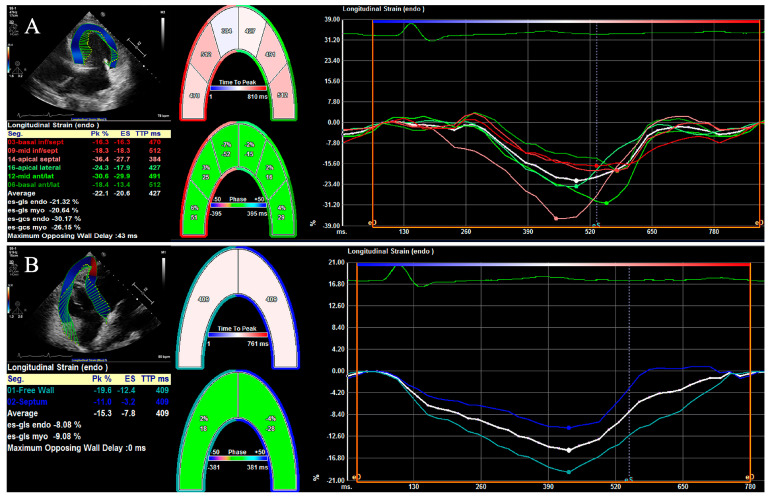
Longitudinal strains of left and right ventricles using two-dimensional speckle tracking echocardiography. (A) Longitudinal strains of left ventricle for each segment and left ventricular global longitudinal strain. (B) Longitudinal strain of right ventricular free wall and septum.
